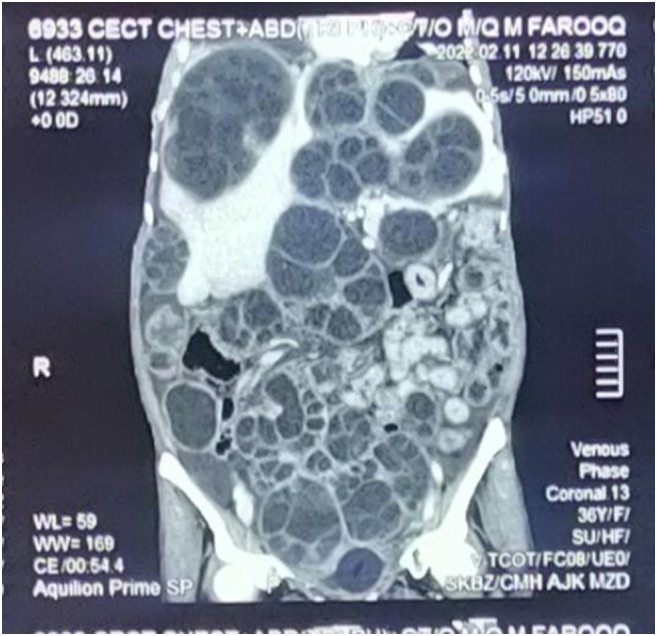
Longitudinal sections of CT scan.
